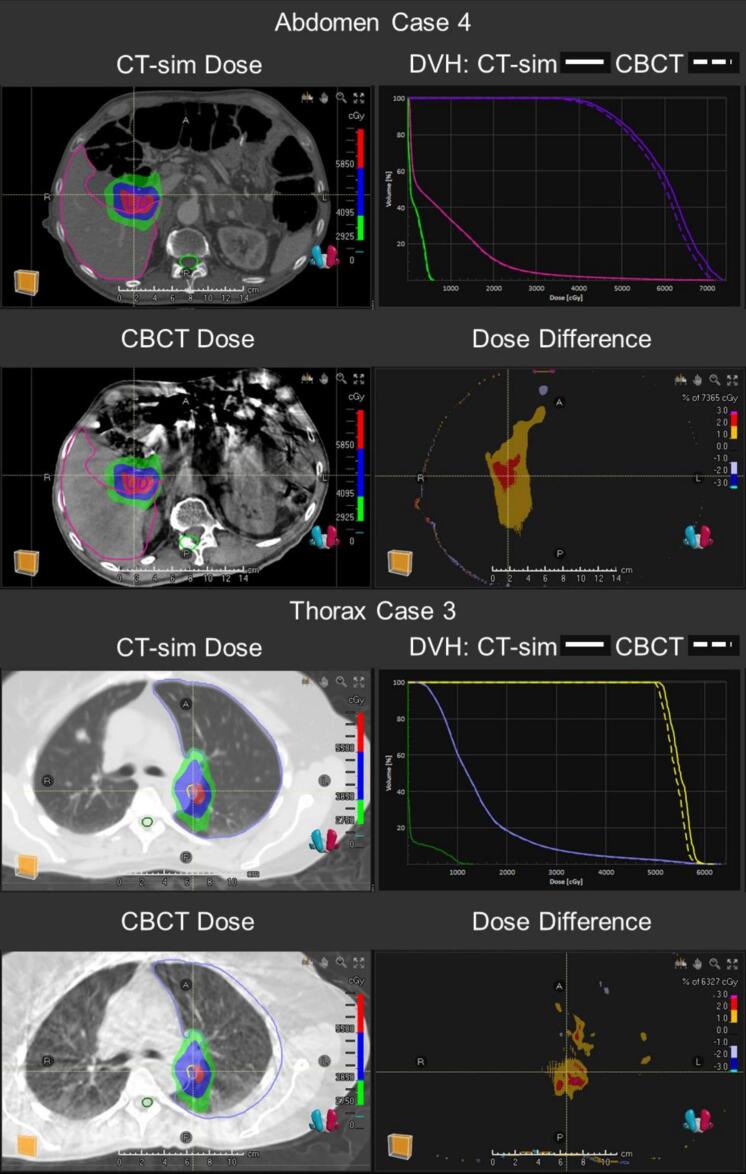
Two worst-case target DVH metric discrepancies caused by the differences in internal anatomy between CT-sim and CBCT in abdomen case 4 (V100%=-7.0%) and thorax case 3 (V100%=-27.4%).
